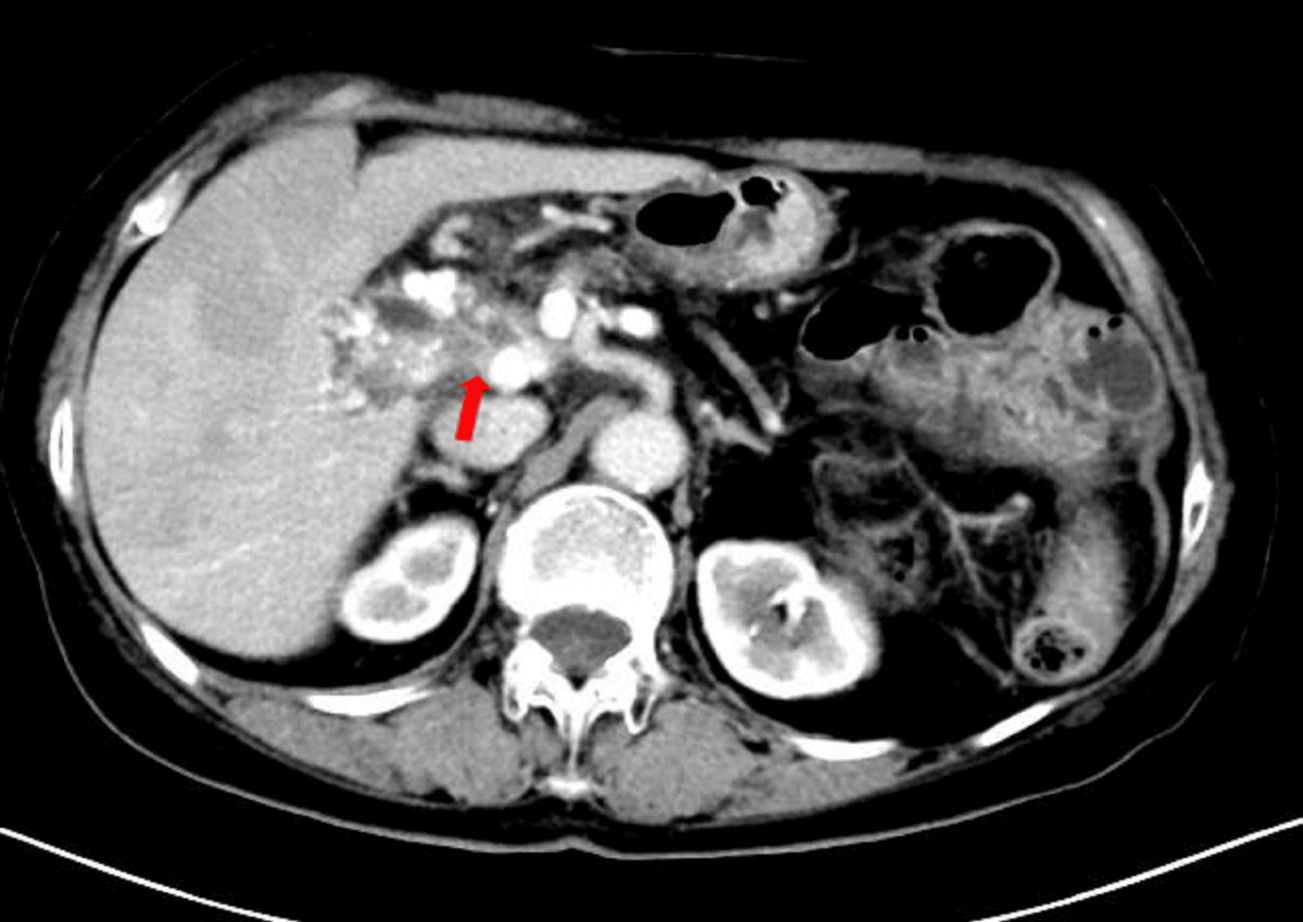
Follow-up CT in January 2024, showing continued reduction in pancreatic head tumor size, assessed as PR (red arrow) CT: computed tomography; PR: partial response
